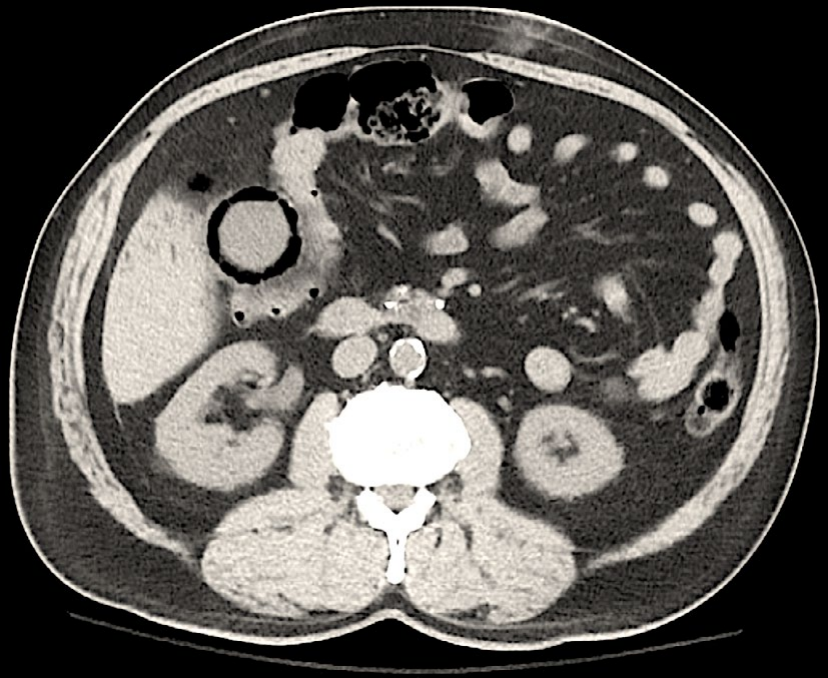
Cross‐sectional CT image of abdomen and pelvis showing air within gall bladder wall, a typical sign of emphysematous cholecystitis. Also noted are chronic calcific pancreatitis changes
